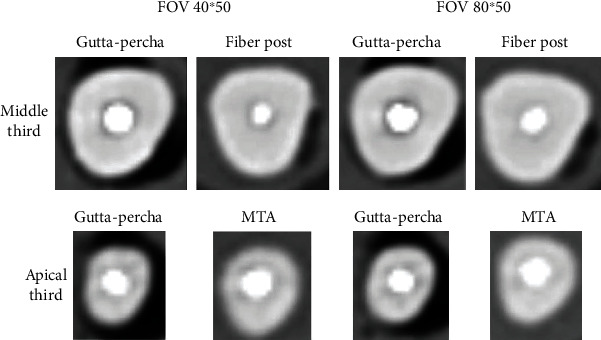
Samples of middle and apical third CBCT images using both fields of view.
